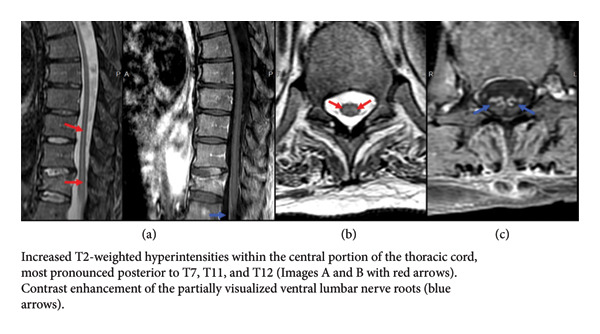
MRI thoracic spine with and without contrast, sagittal (image (a)) and axial views (images (b) and (c), respectively). Increased T2‐weighted hyperintensities within the central portion of the thoracic cord, most pronounced posterior to T7, T11, and T12 (Images (a) and (b) with red arrows). Contrast enhancement of the partially visualized ventral lumbar nerve roots (blue arrows).
